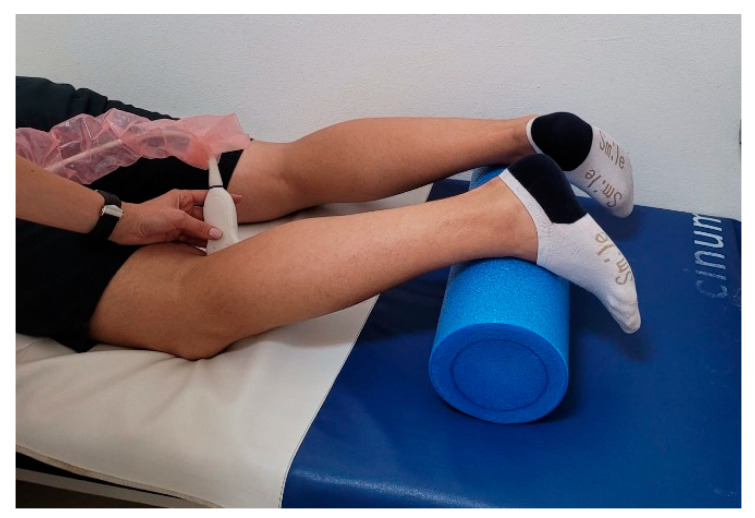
Patient position during ultrasound assessment.
